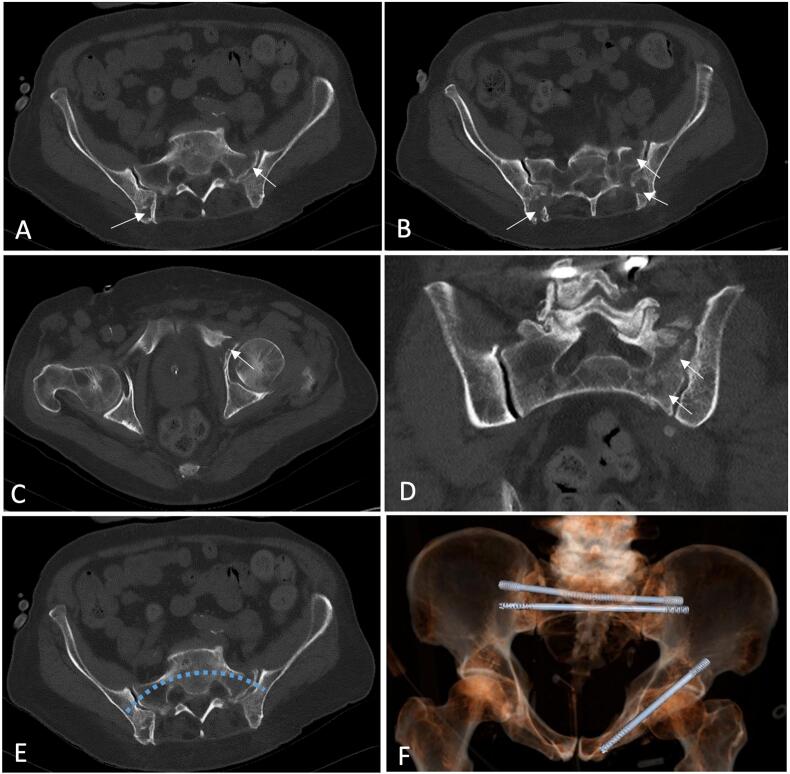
A-D show representative axial CT-scan reconstructions with the fracture lines and bone stock loss marked by the arrows. E shows an axial view with a the planned implant corridor in the S1 body, F shows a 3D-reconstruction with preoperative implant planning (3D-Trauma, Sectra, Sweden). Screw templates were used to mimic the corridors for the photodynamic nail implants, therefore two oblique 8.0 mm corridors were planned to mimic the arc-like configuration of the implant Additionally a transiliosacral corridor was planned in S2 and a antegrade corridor for the left superior pubic ramus was planned.
